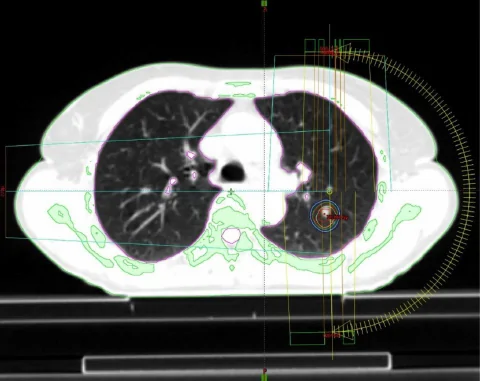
Stereotactic body radiotherapy planning for pulmonary metastasis. Treatment planning computed tomography scan of the thorax demonstrating a metastatic nodule in the right lung (target volume marked with concentric isodose lines). Dose distribution was achieved using volumetric modulated arc therapy (VMAT)-based stereotactic body radiotherapy, delivering 60 Gy in three fractions with precise target coverage and sparing of the surrounding normal lung tissue.
